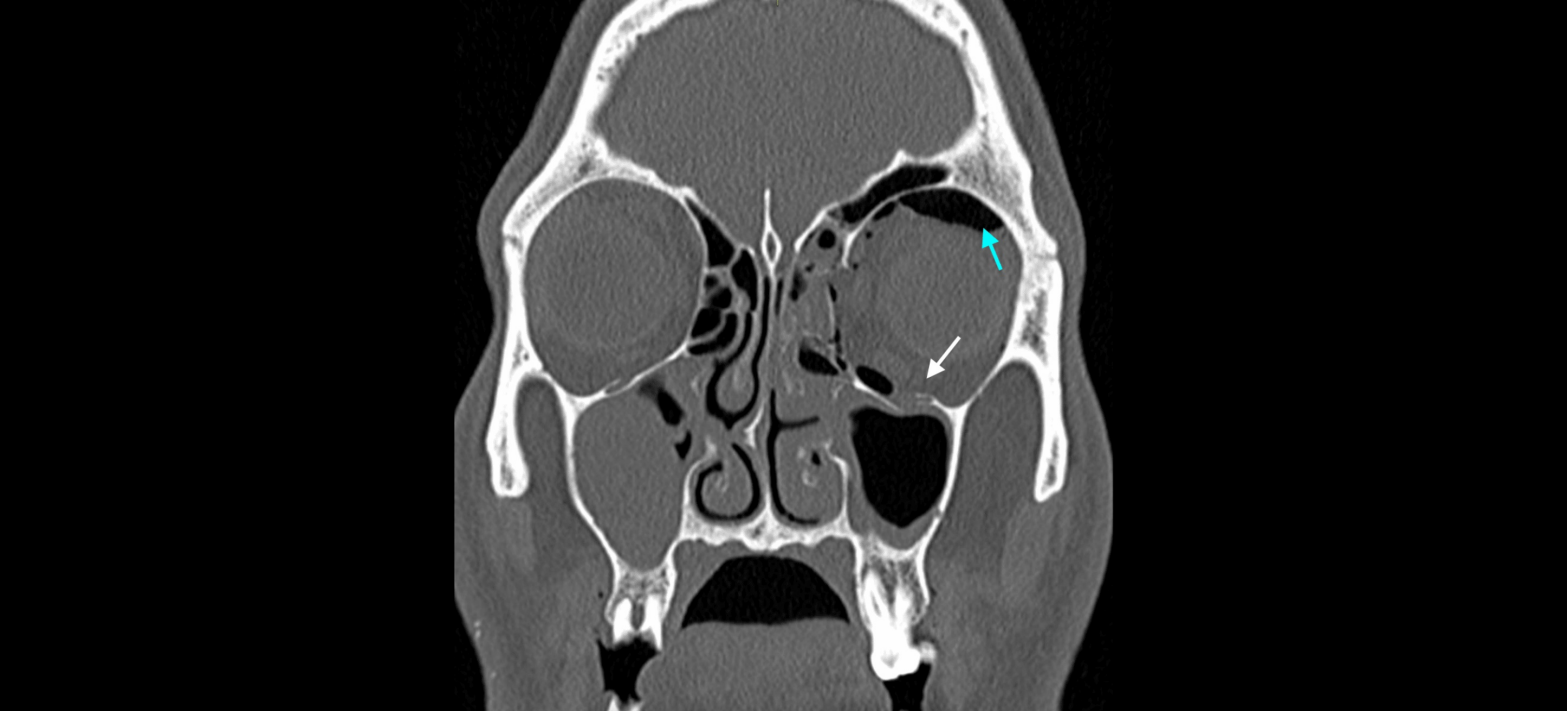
Coronal CT of the facial bones Coronal CT acquisition with bone algorithm showing a left orbital floor fracture with herniation of soft tissue into the maxillary sinus (white arrow) and the presence of intraorbital emphysema (blue arrow), a subtle sign of fracture.
